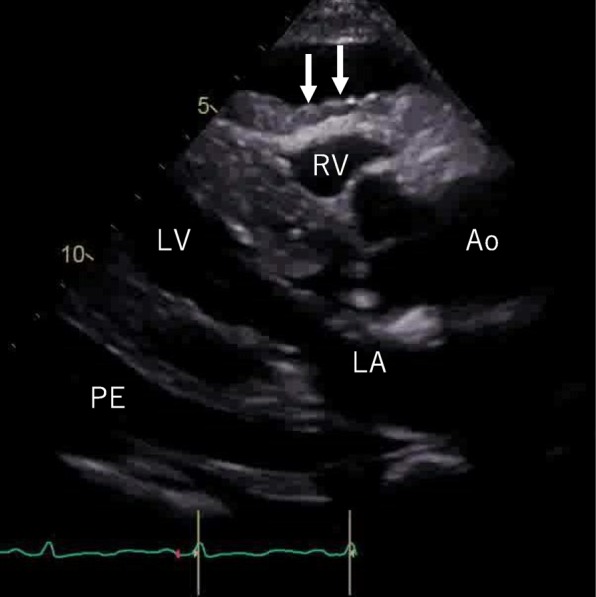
Echocardiography on day 9 (diastole). Pericardial effusion up to 10 mm appeared behind the left ventricle inferior-lateral lesion, leading to the collapse of the right ventricle (white arrows) (LA, Left Atrium; LV, Left Ventricle; RV, Right Ventricle; Ao, Aorta; PE, Pericardial Effusion)
